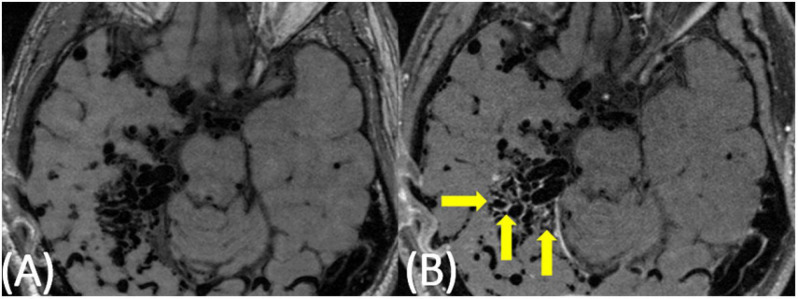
51-year-old man with a large AVM centered in the right temporoparietal lobe. On pre-contrast VW-MRI (A), no intrinsic T1 hyperintensity is present. On post-contrast VW-MRI (B), there were multiple areas of hyperintensity at the vessel wall within the nidus (yellow arrows).
